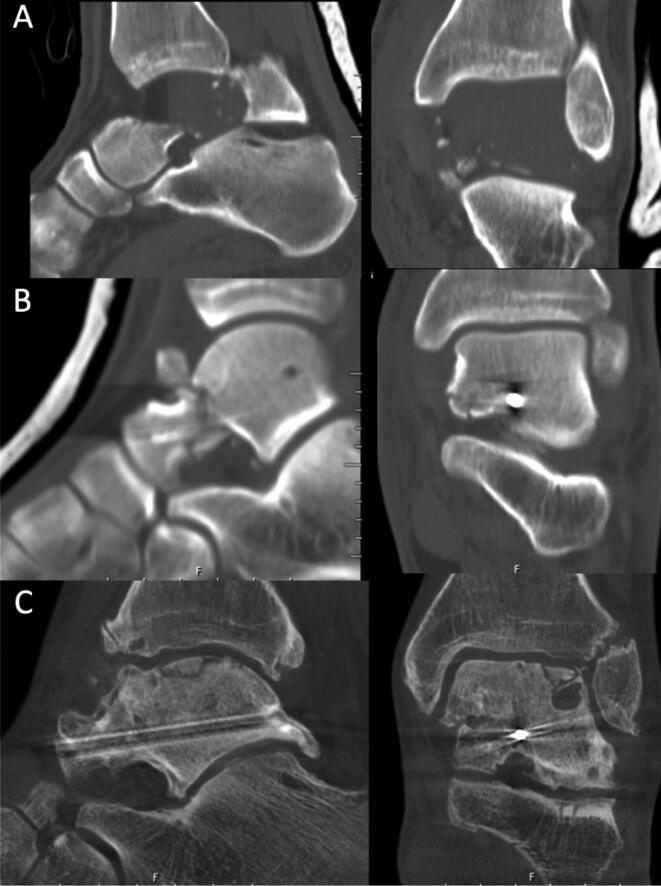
CT of a Hawkins type 3 fracture (A) in a 26-year-old man after a fall from a ladder. The fracture was treated with screw fixation (B), a non-weight-bearing cast for 8 weeks, and alendronate for 6 months. After 12.5 years, the fracture appeared to be healed on CT, showing post-traumatic talocrural OA and partial collapse of the talar dome (C).
